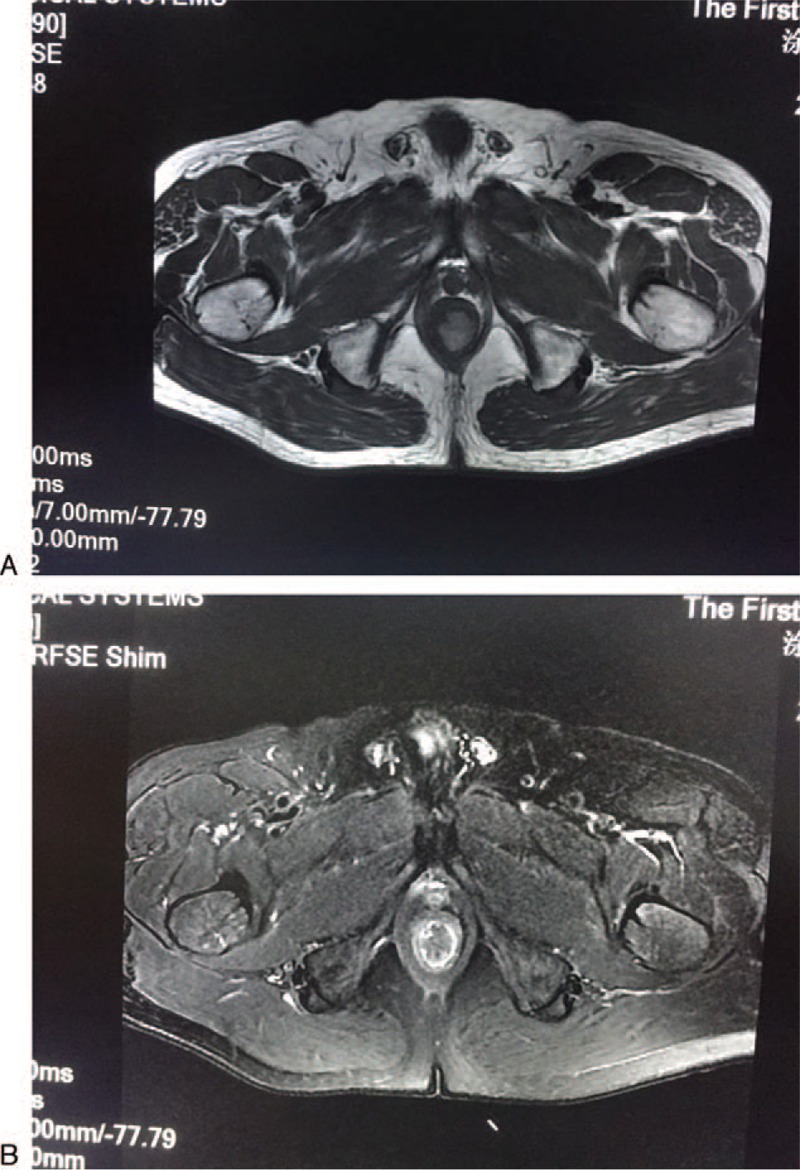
Magnetic resonance imaging showing a mass in the anal region. A: The tumors yielded high signal intensity on T1-weighted axial image; B: Low intensity was produced on the T2-weighted axial image.
